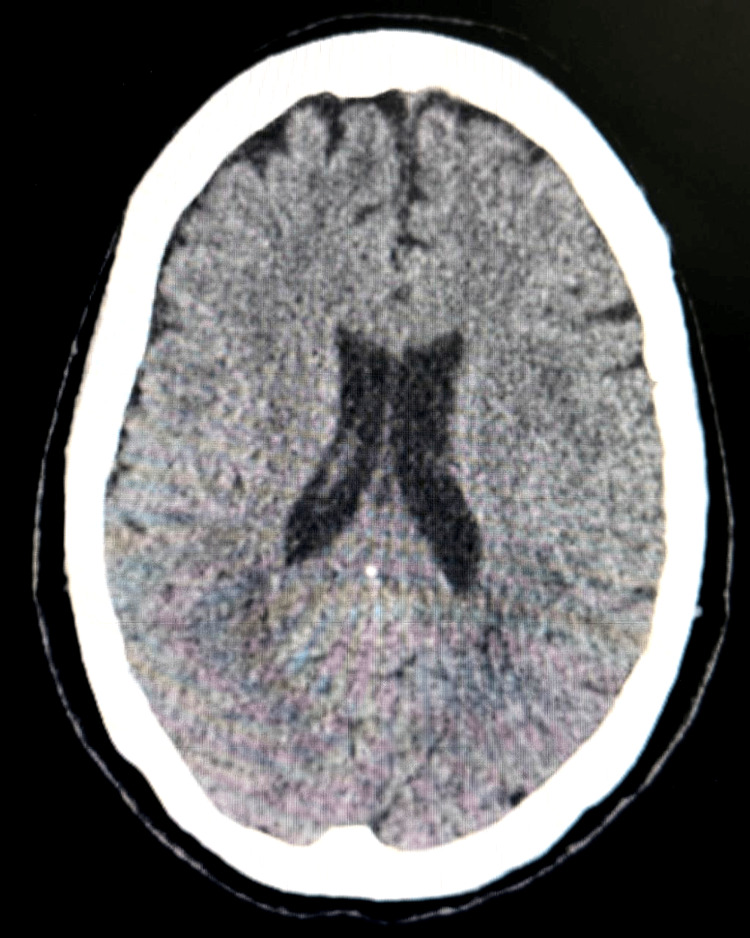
Non-contrast CT of the brain Axial non-contrast CT showing preserved gray-white matter differentiation and symmetric ventricular system, without evidence of acute hemorrhage, mass effect, or ischemic lesions.
